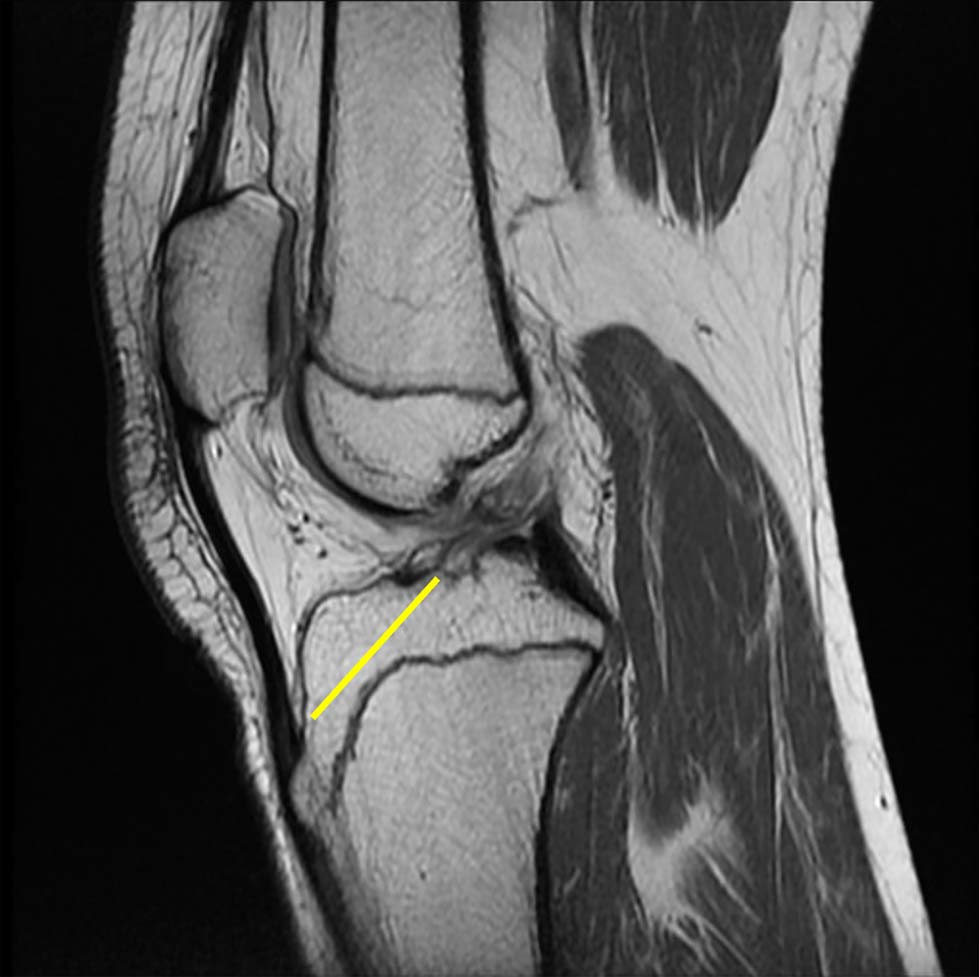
Sagittal T1-weighted MRI showing the knee of a 13-year-old boy, measuring of the maximum oblique intraepiphyseal depth.
